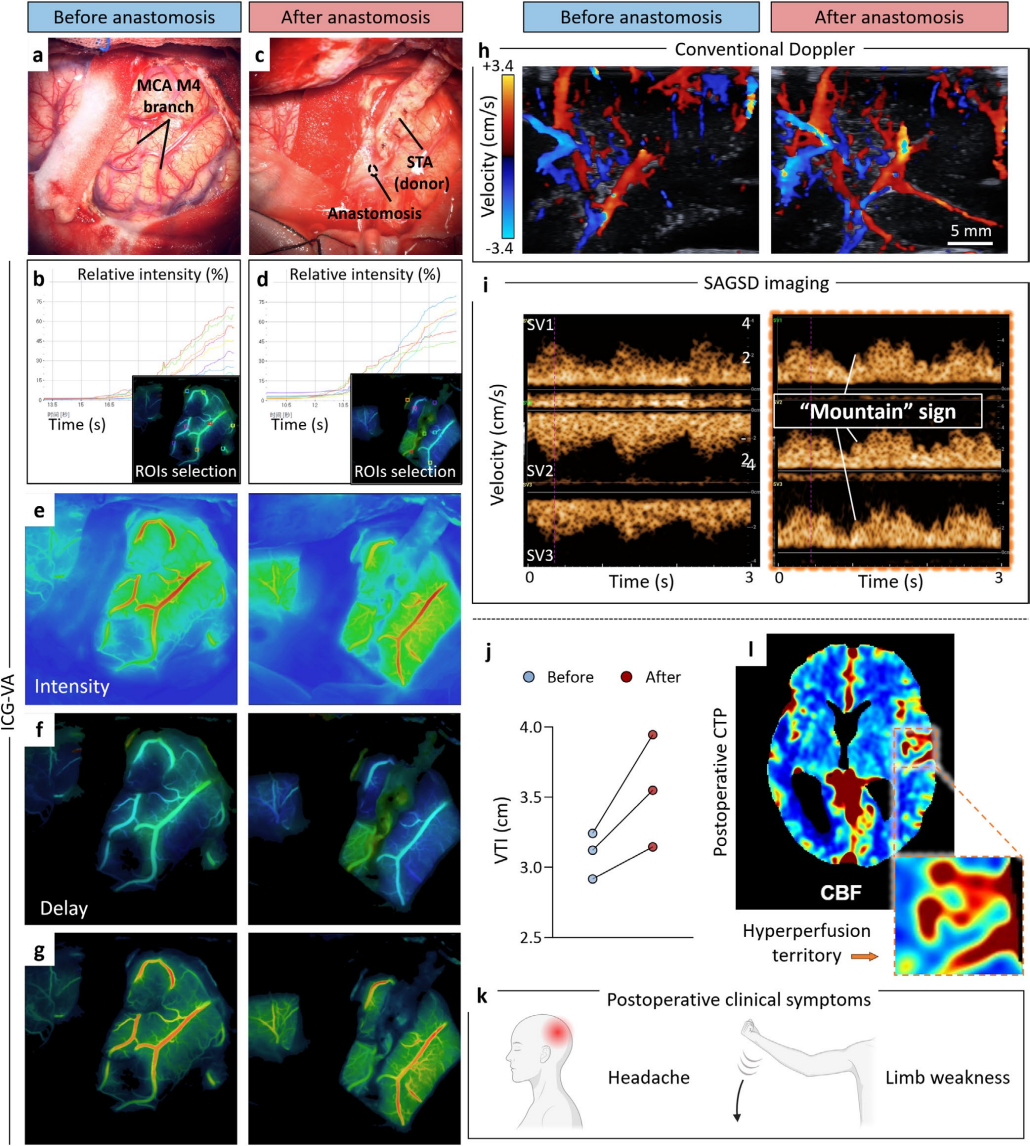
A representative case of evaluating intraoperative hemodynamics in CHS patients using different modalities. A 49‐year‐old woman with moyamoya disease underwent STA‐MCA bypass (a, c). The time–intensity curves of ICG‐VA (b, d) intensity (e), delay (f), and time to peak (g) maps of the brain surface indicated unchanged after anastomosis, and conventional Doppler merely demonstrated augmented flow signals (h). SAGSD interrogation of subcortical hemodynamics, however, revealed the characteristic “mountain” spectral pattern post‐revascularization (i), with velocity‐time integral (VTI) rising from 3.09 (mean) to 3.55 (mean) cm (j). CBF mode of postoperative CTP disclosed a focal hyperperfusion territory (highlighted and enlarged) beneath the left frontal cortex (k), indicating CHS. On post‐operative day 2, this patient developed a headache and contralateral limb weakness (l). Created with BioRender.com. CBF, cerebral blood flow; CHS, cerebral hyperperfusion syndrome; CTP, computed tomography perfusion; ICG‐VA, indocyanine green videoangiography; ROI, region of interest; SAGSD, synchronous arbitrary gate spectral Doppler; STA‐MCA, superficial temporal artery to middle cerebral artery; SV, sample volume.
